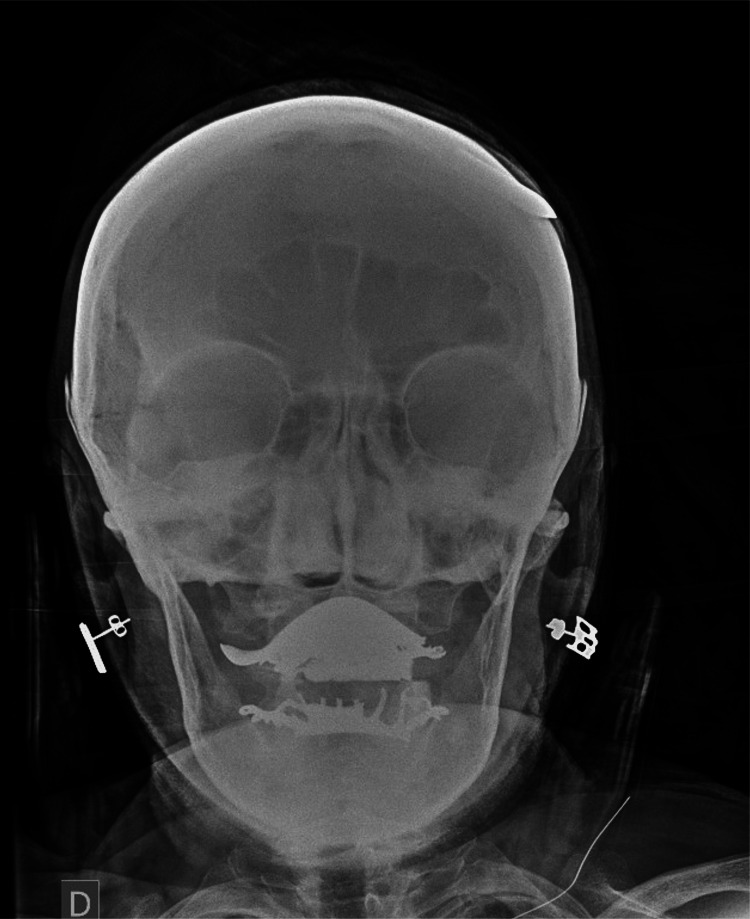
Frontal cranial X-ray. The arrow tip in apparent contact with or partially penetrating the outer cortical bone of the skull.
